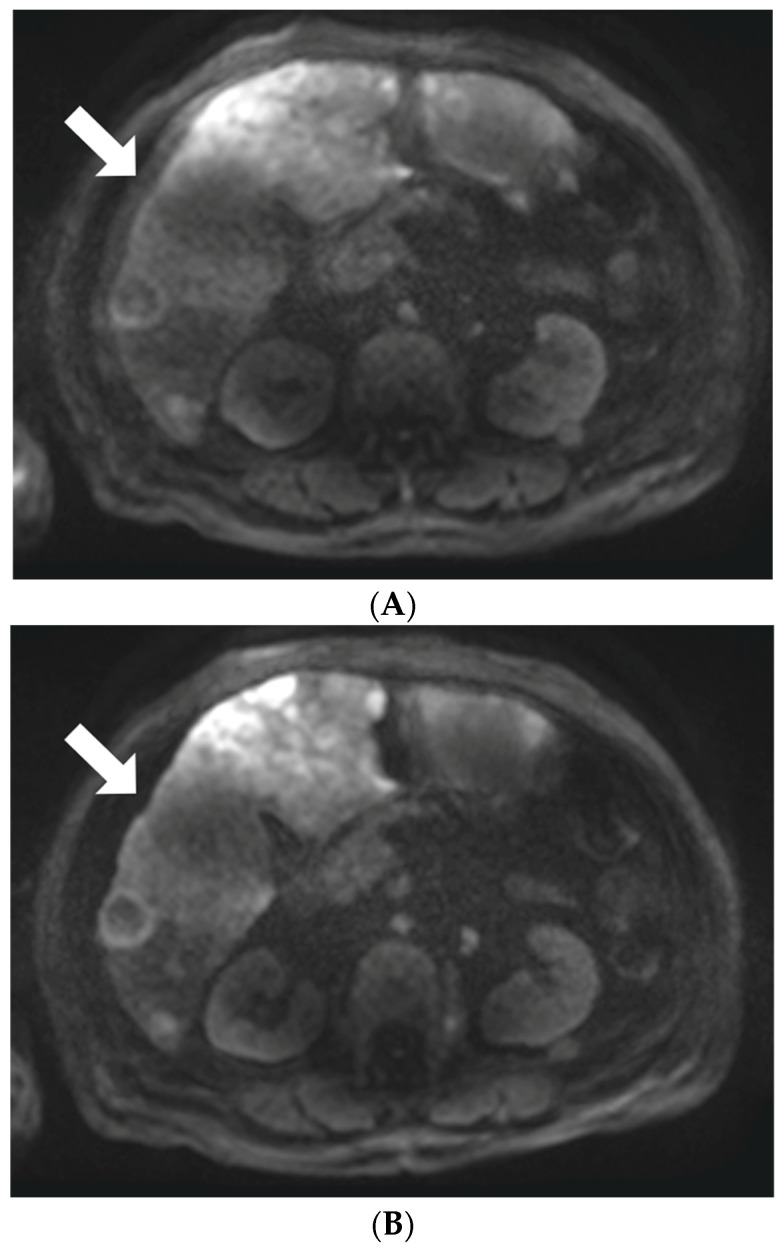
A 72-year-old woman with intrahepatic cholangiocarcinoma who underwent diffusion-weighted imaging (DWI). (A) Axial conventional c-DWI (b = 800 s/mm^2^) and (B) axial motion-corrected (moco) DWI ((b = 800 s/mm^2^). Margin sharpness of the right liver lobe (arrows) is superior in moco-DWI (B) to that in c-DWI (A).
